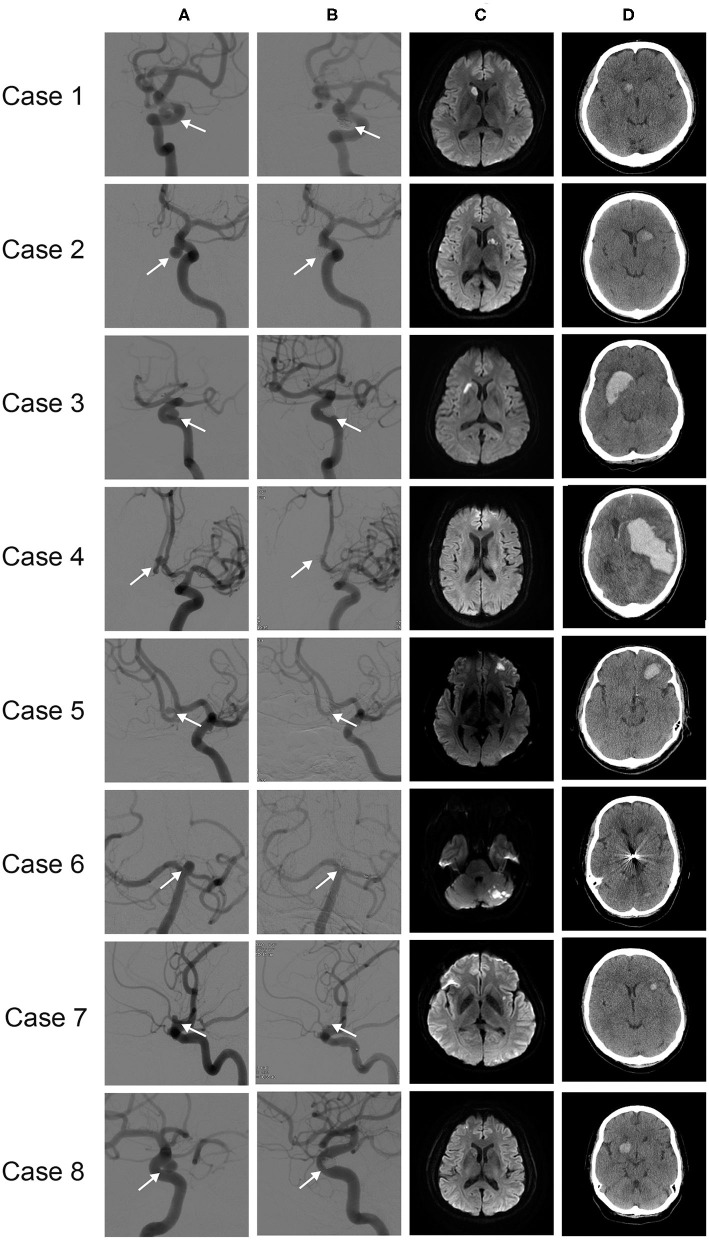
Eight cases of d-ICH: (A) pre-procedural angiograms, (B) post-procedural angiograms, with treated aneurysm indicated (arrow), (C) one-day post-procedural follow-up DWI, (D) follow-up CT scan, d-ICH identified.
